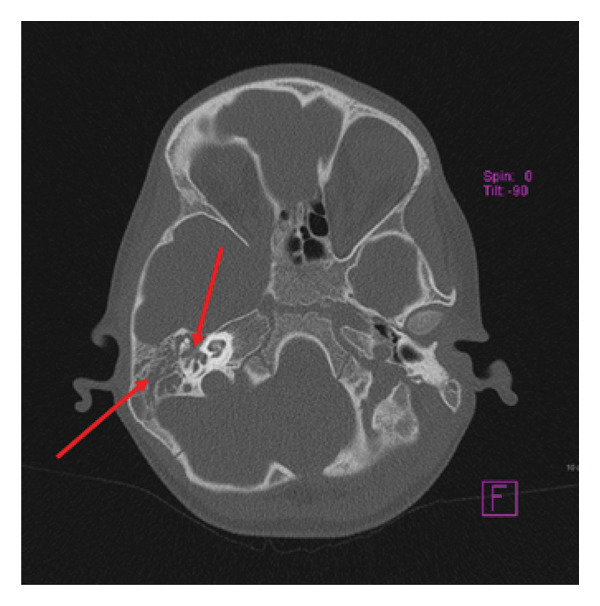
Temporal bone CT‐filled mastoid cells with pathological substrate, thinning and destruction of septa, and involvement of the middle ear cavity (imaging findings).
